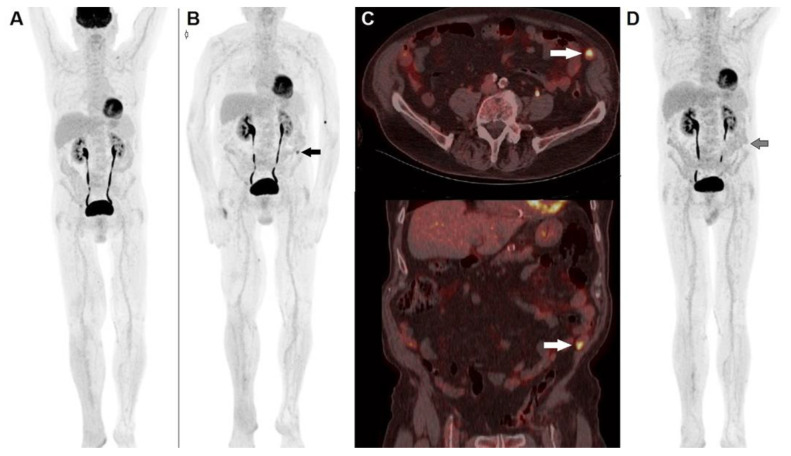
A 74-year-old male, previously submitted to excision of nodular cutaneous melanoma of the right foot (Breslow thickness of 8 mm, Clark level IV, stage pT4a), performed ^18^F-FDG PET/CT before the start adjuvant immunotherapy. (A) MIP image showed physiological tracer biodistribution, with no evidence of pathological accumulation. PET/CT MIP (B) performed after 3 months of PD-1 blocker (nivolumab) depicted the appearance of an area of increased tracer accumulation in the left iliac fossa (black arrow). (C) Fused corresponding PET/CT axial (upper row) and coronal (lower row) of the abdominal region demonstrated the onset of a hypermetabolic nodule next to the abdominal wall, suspected to be peritoneal localization (white arrow). The pattern was interpreted according to PERCIMT criteria (i.e., pseudo-progression) and the patient continued immune check-point inhibitor. A further PET/CT MIP (D) after 6 weeks demonstrated complete spontaneous regression of the area of increased ^18^F-FDG accumulation in the left iliac fossa (black arrow), thus confirming the diagnosis of pseudo-progression.
